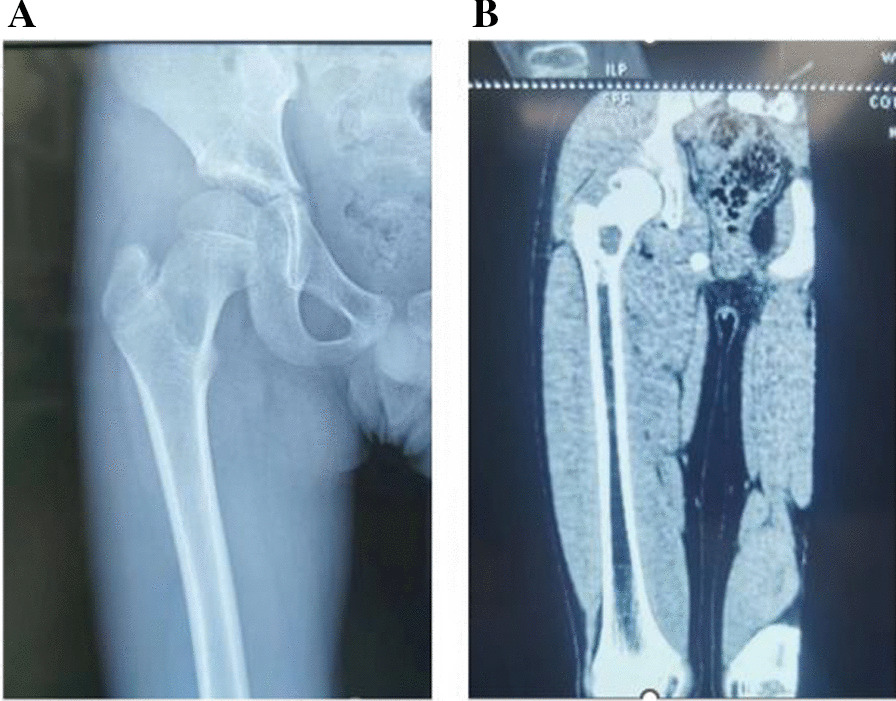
Imaging findings of femoral neck lesions: CT (A) and X-ray (B) findings of eosinophilic granuloma
